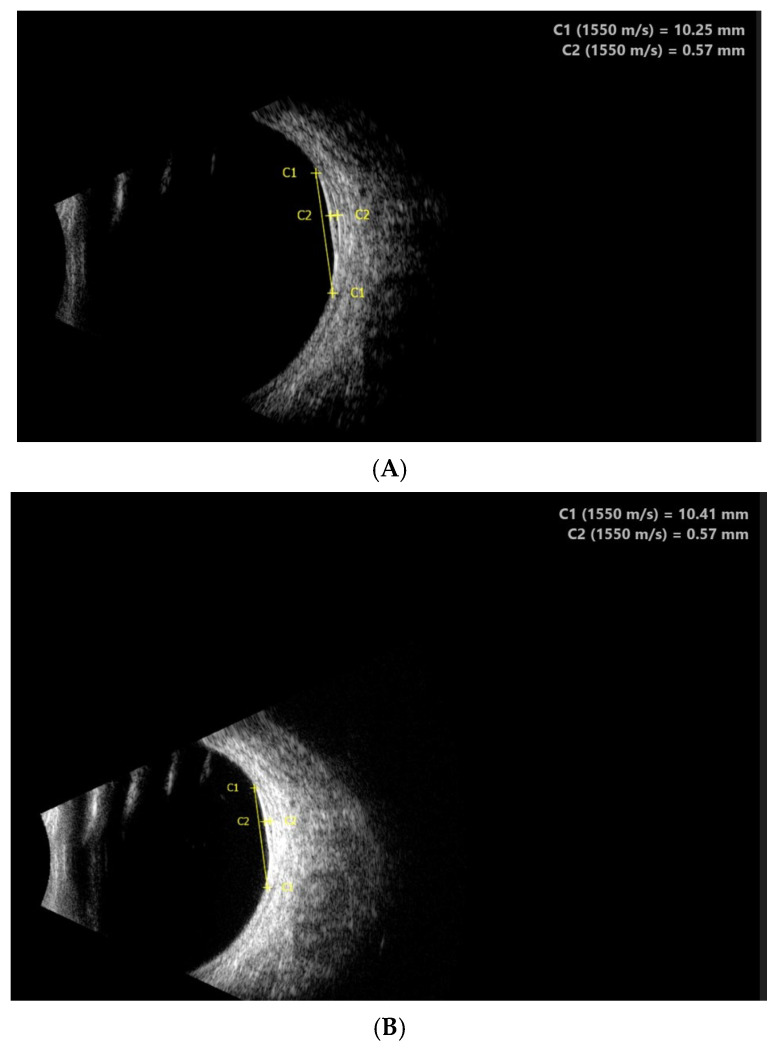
B-ultrasound of the choroidal nevus—at the time of diagnosis (A) and after 1 year (B).
